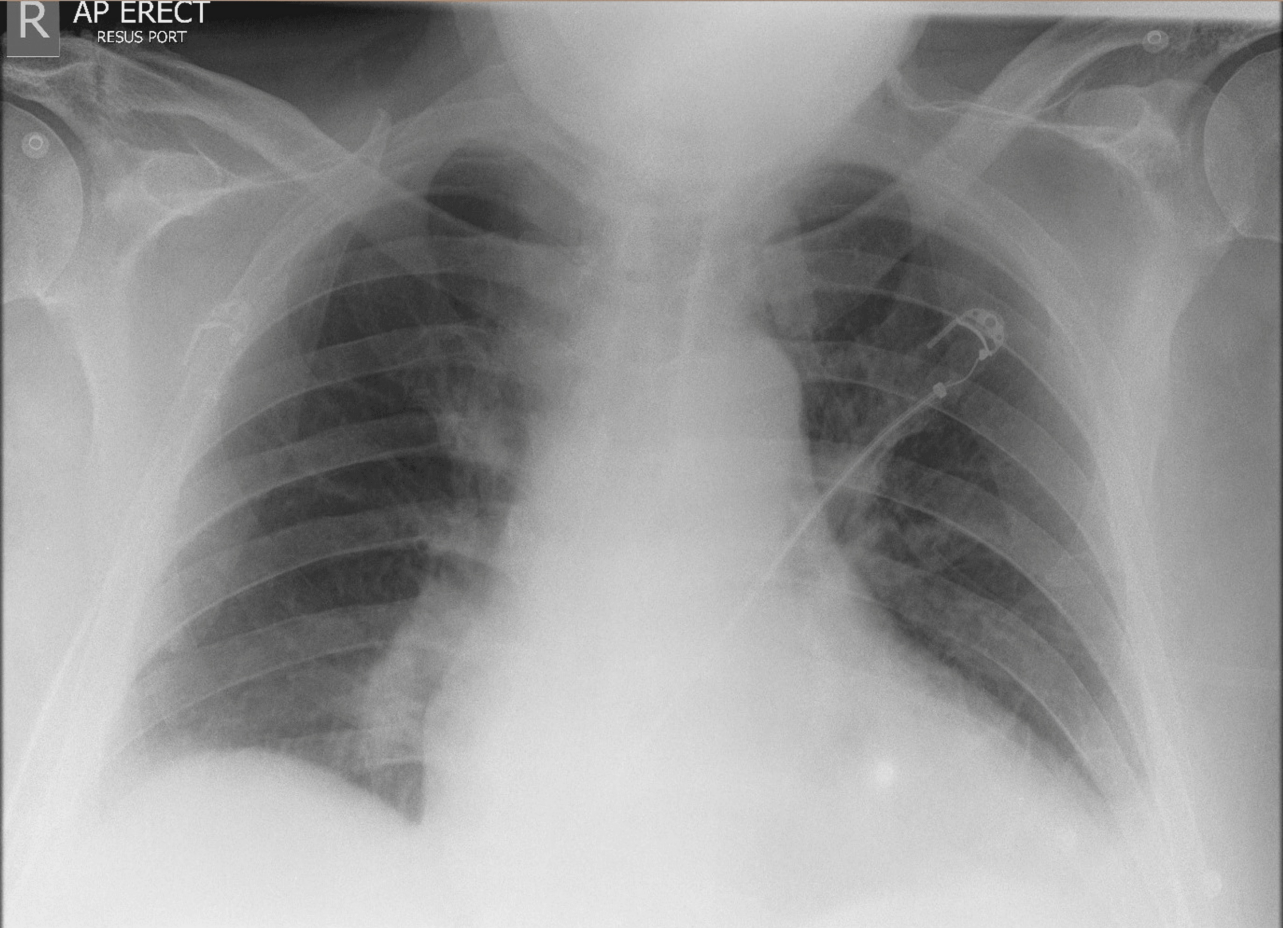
Chest X-ray of the patient
